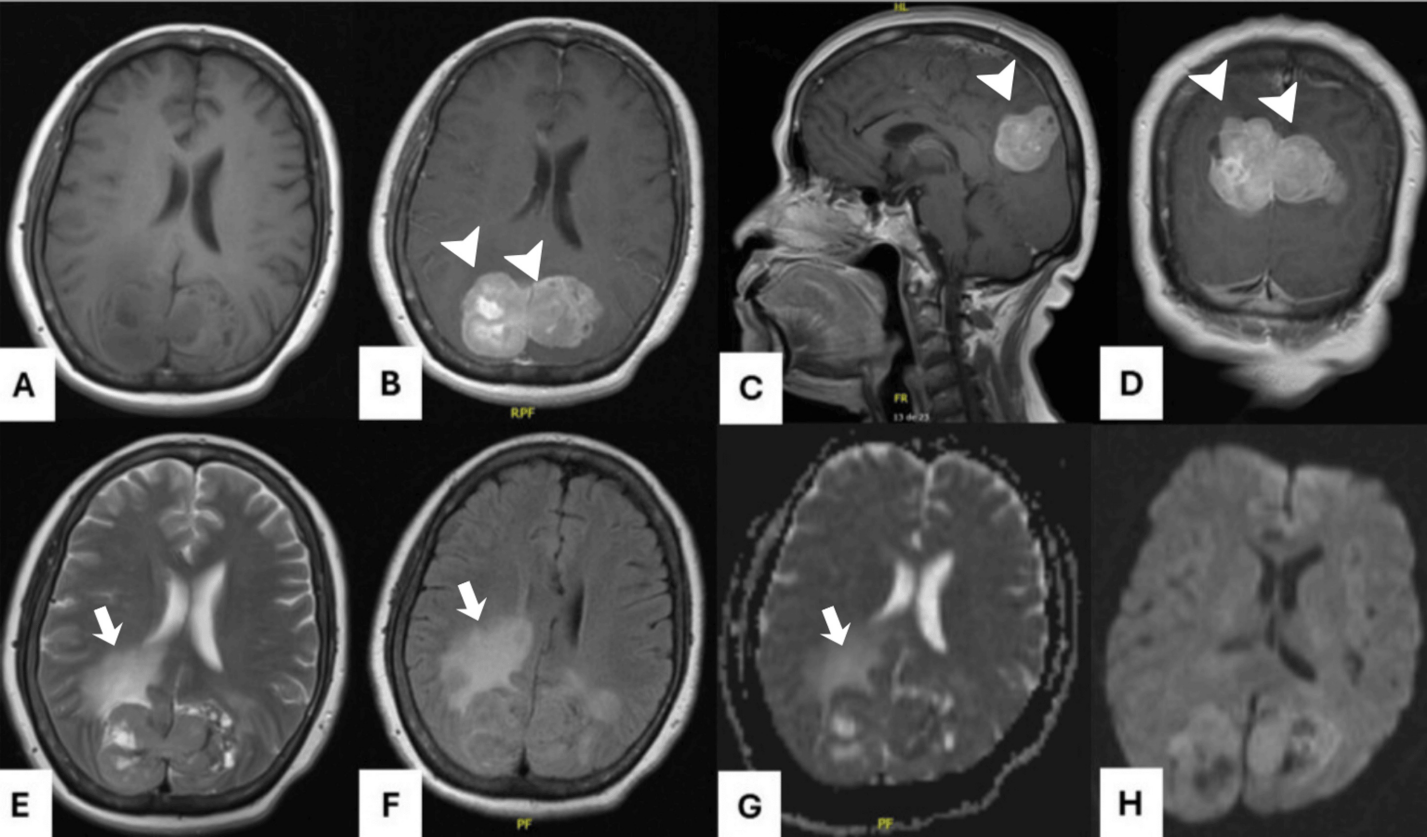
Bilateral lesion in the posterior third of the falx. The lesion appears isointense on (A) T1, (E) T2, and (F) FLAIR sequences, with a lobulated morphology and well-defined borders, presenting heterogeneous central areas. (B, C, D) Significant enhancement was observed after contrast administration. (G) On ADC, it appears predominantly hypointense, with some hyperintense areas. (H) The inverse of what is observed on DWI. Arrowheads: Enhancing lesion, Arrows: Peritumoral edema. FLAIR: fluid-attenuated inversion recovery; ADC: apparent diffusion coefficient; DWI: diffusion-weighted imaging
